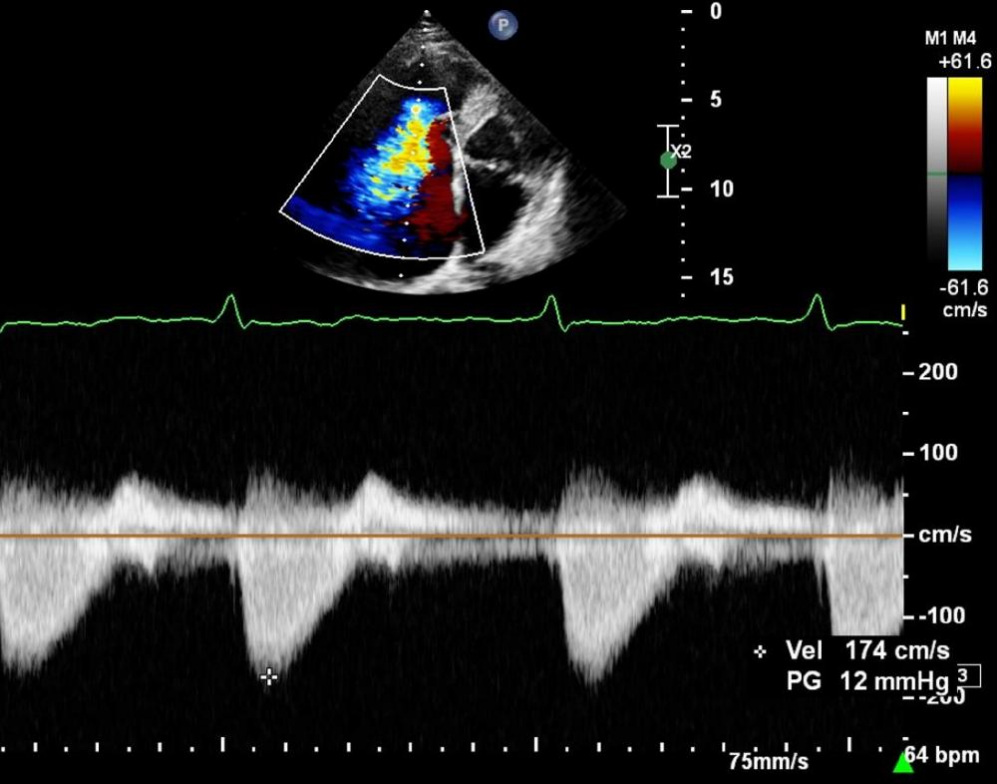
Apical four-chamber view, continuous wave Doppler through the tricuspid valve. Rapid equalization of pressure in an early peaking dense severe tricuspid regurgitation jet.
